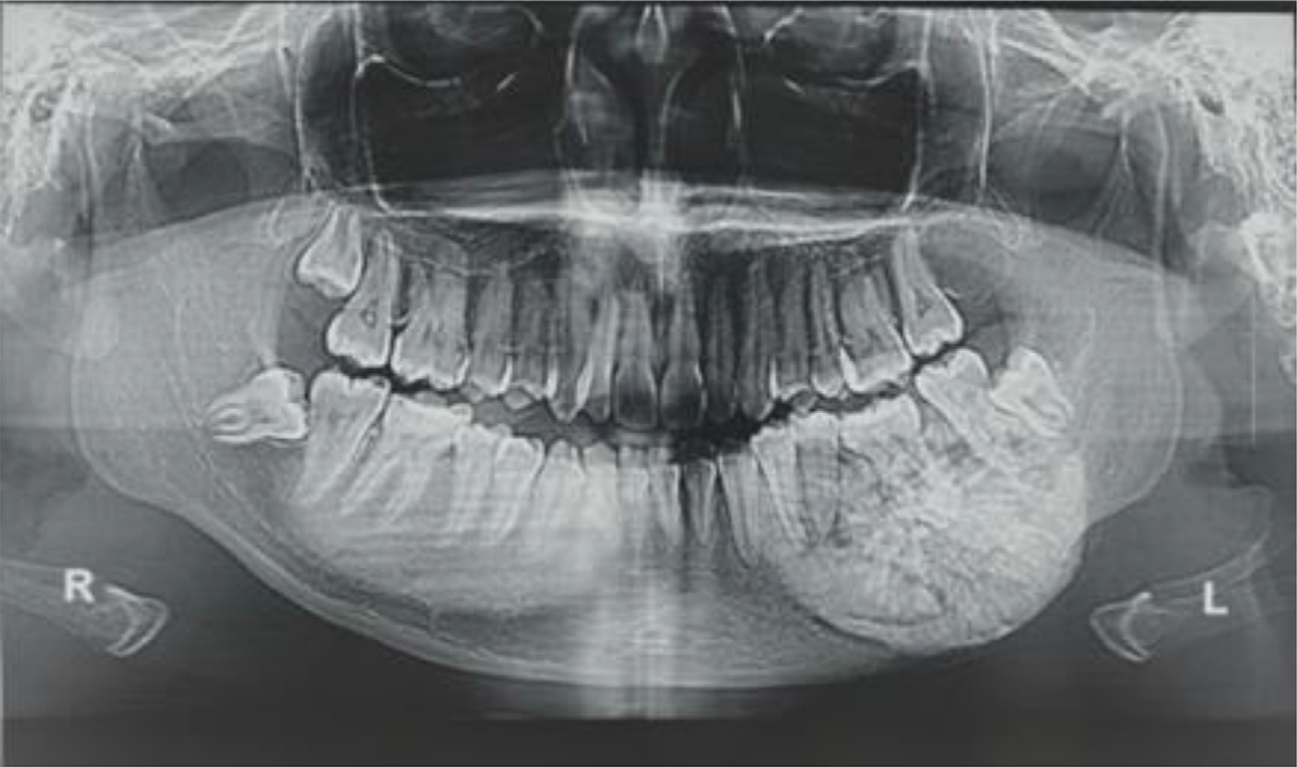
Panoramic radiograph demonstrating a radiopaque mass with well-defined borders, partially delineated by a thin radiolucent rim.
